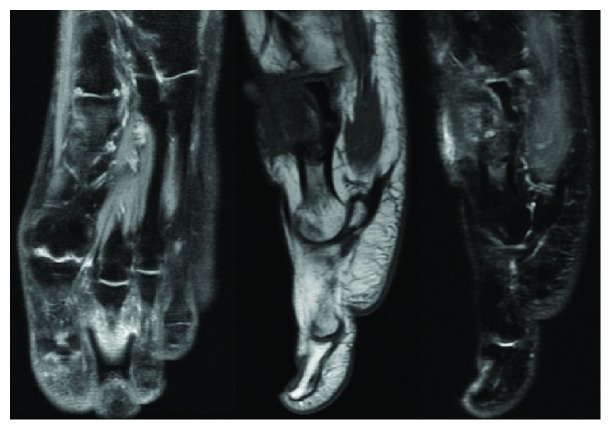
Magnetic resonance imaging showing a large soft-tissue mass surrounding the first metatarsal circumferentially and abutting the second metatarsal with high T2 signal and no T1 signal changes at the base of the second metatarsal.
